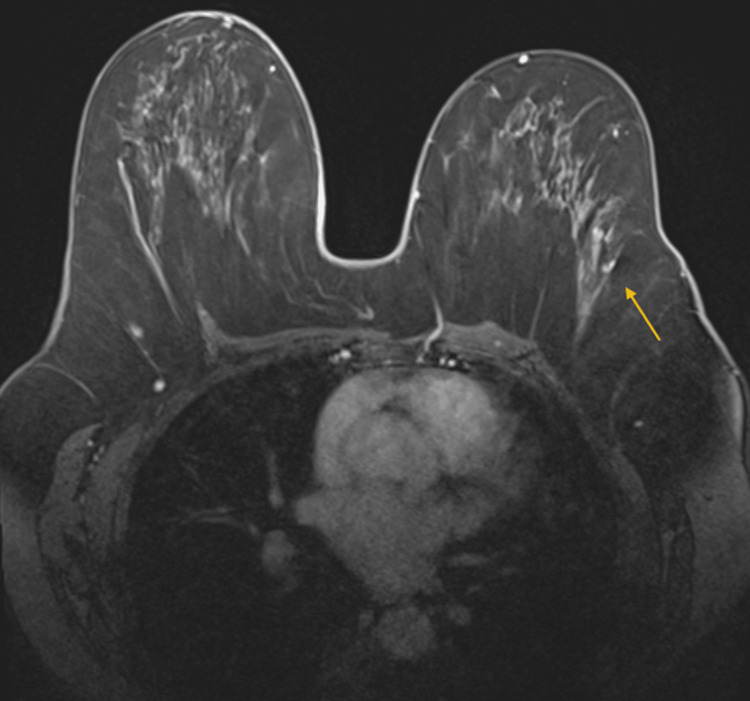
MRI of the breasts showing left breast cancer in the upper outer quadrant (yellow arrow)
